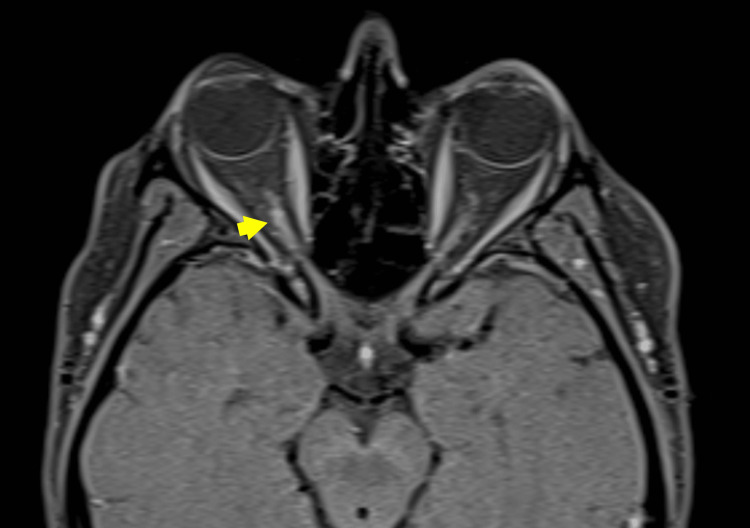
Post-contrast axial T1-weighted orbital MRI with fat suppression showing right optic neuritis. Axial post-contrast T1-weighted image with fat suppression shows abnormal enhancement and mild thickening of the right optic nerve (yellow arrowhead), consistent with acute optic neuritis.
